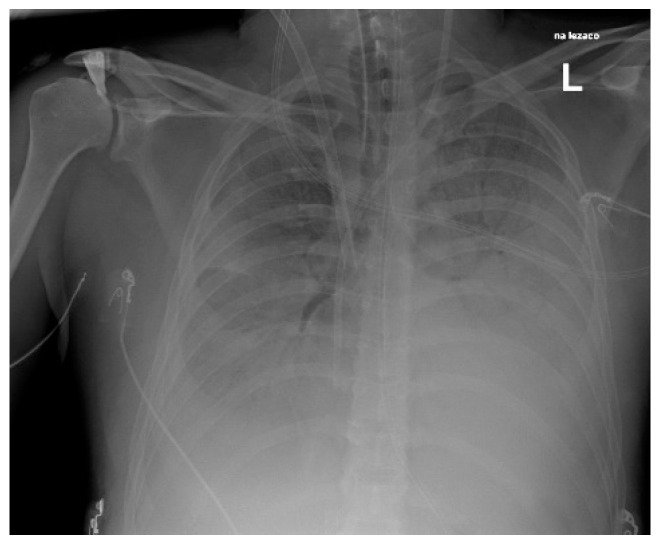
Chest X-Ray. Patient with *S. pyogenes* and AH1N1 pneumoniae.
